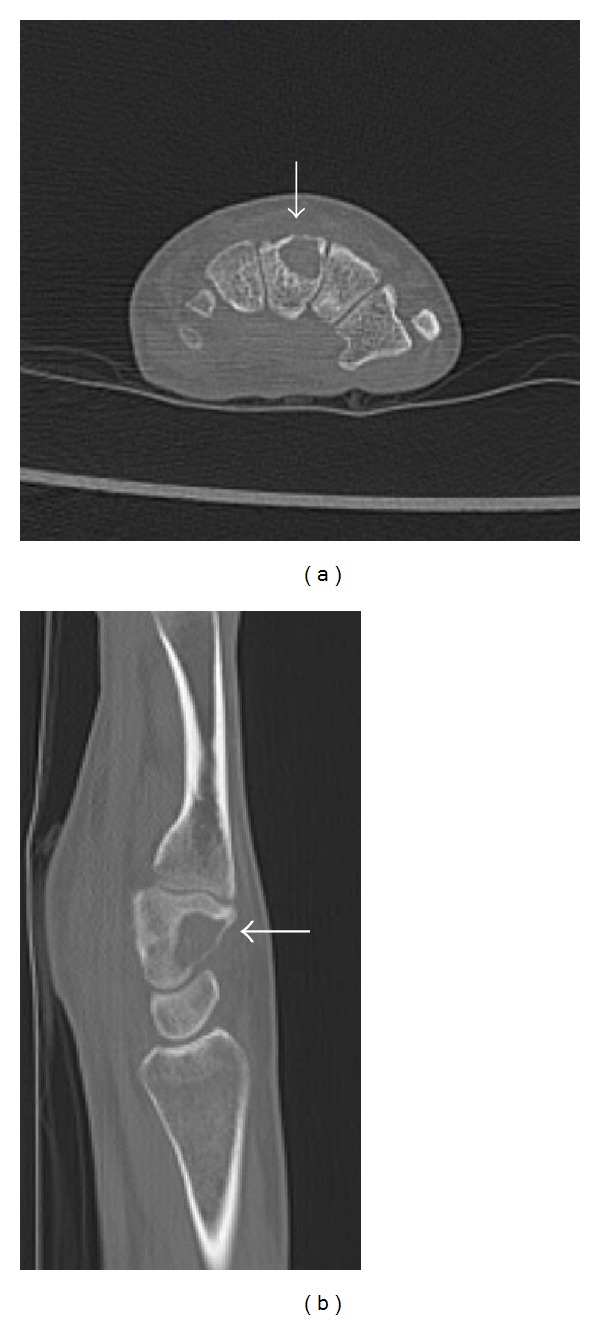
CT images of the patients. Cystic lesion with sclerotic margin is seen in axial plane and sagittal plane ((a) and (b), resp.). White arrows are showing the cystic lesion.
